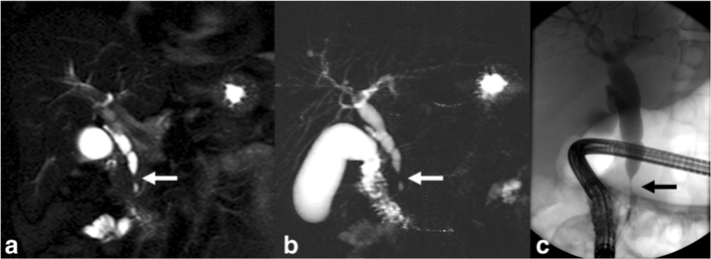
Distal CCA. Common bile duct stricture due to grade 4 invasive carcinoma. Coronal T2-weighted image (a) and MRCP (b) image showing a short segmental narrowing (arrow) with proximal dilatation. ERCP (c) showing a short segmental stricture representing the invasive CCA.
